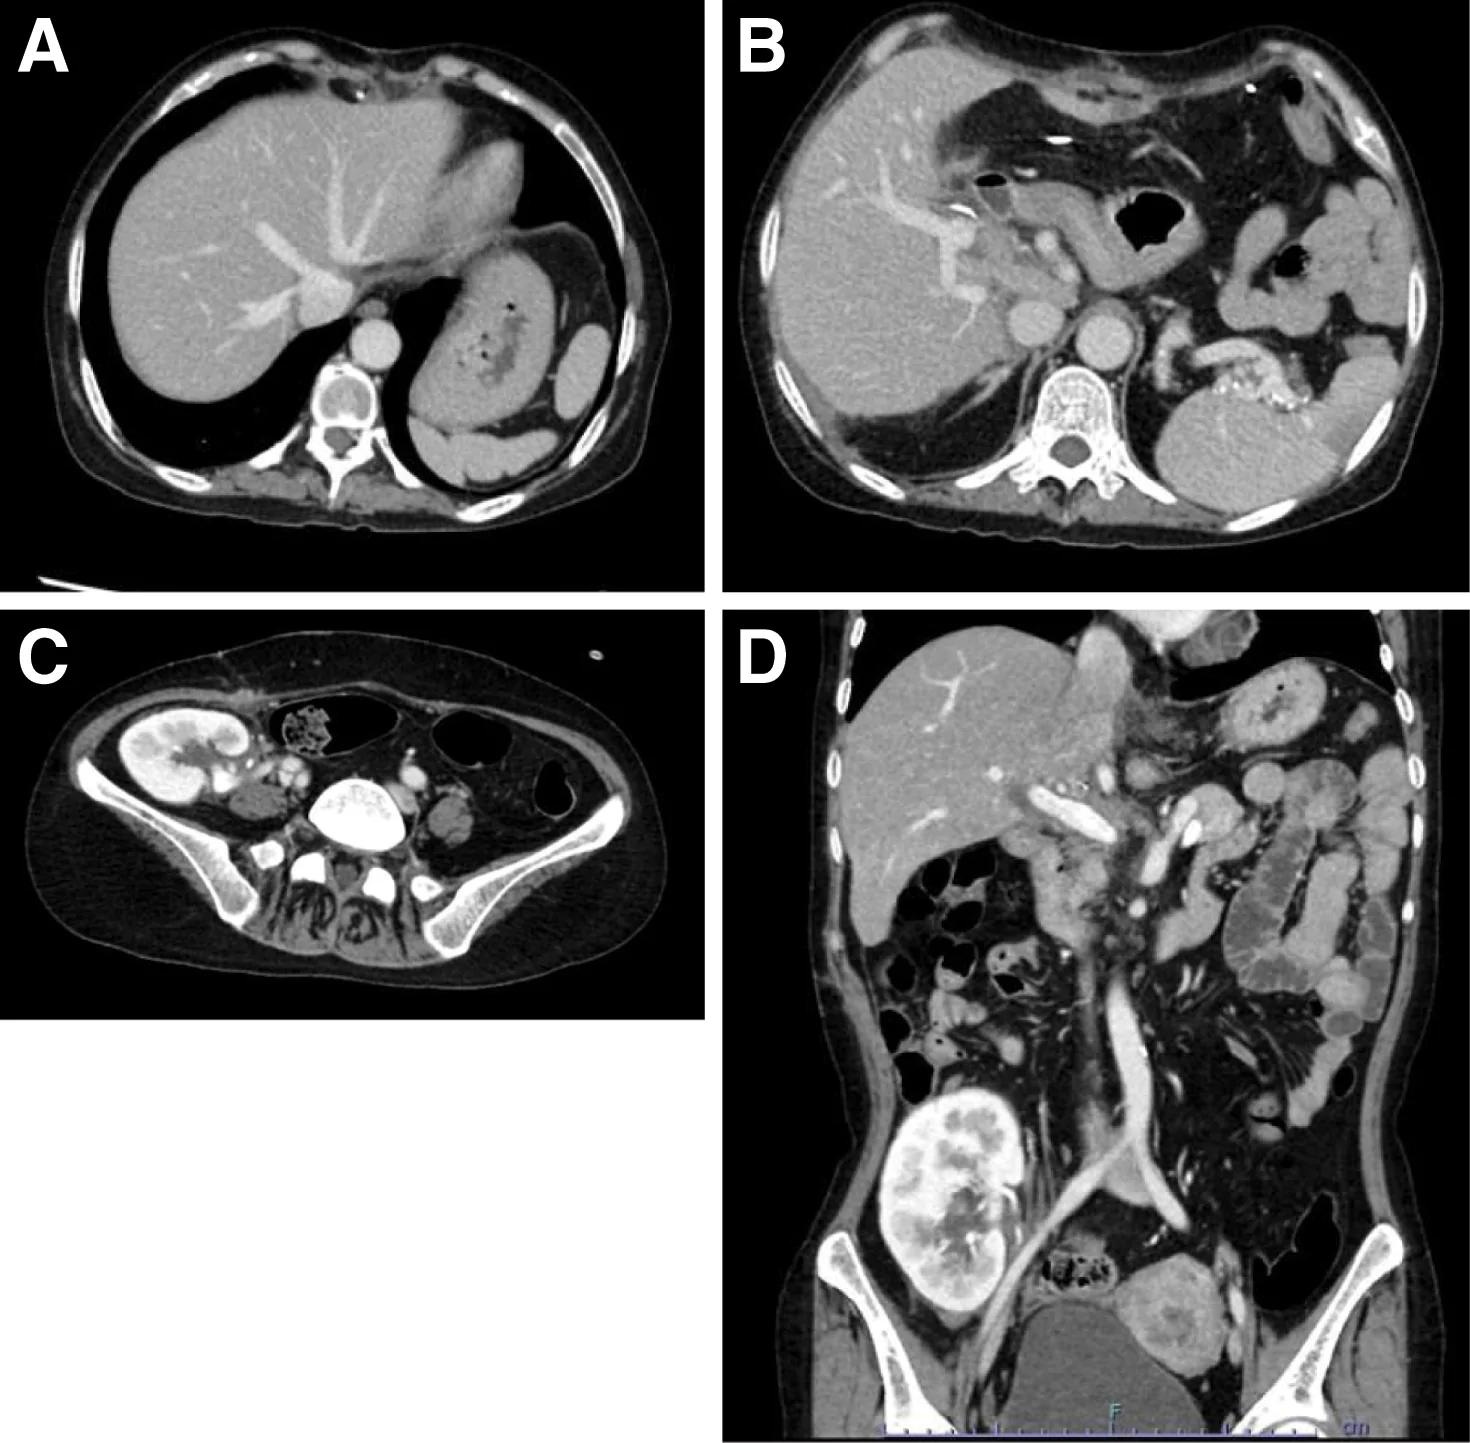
The CT findings of the liver and kidney after the SLKT (A, B, C and D). SLKT, simultaneous liver-kidney transplantation
